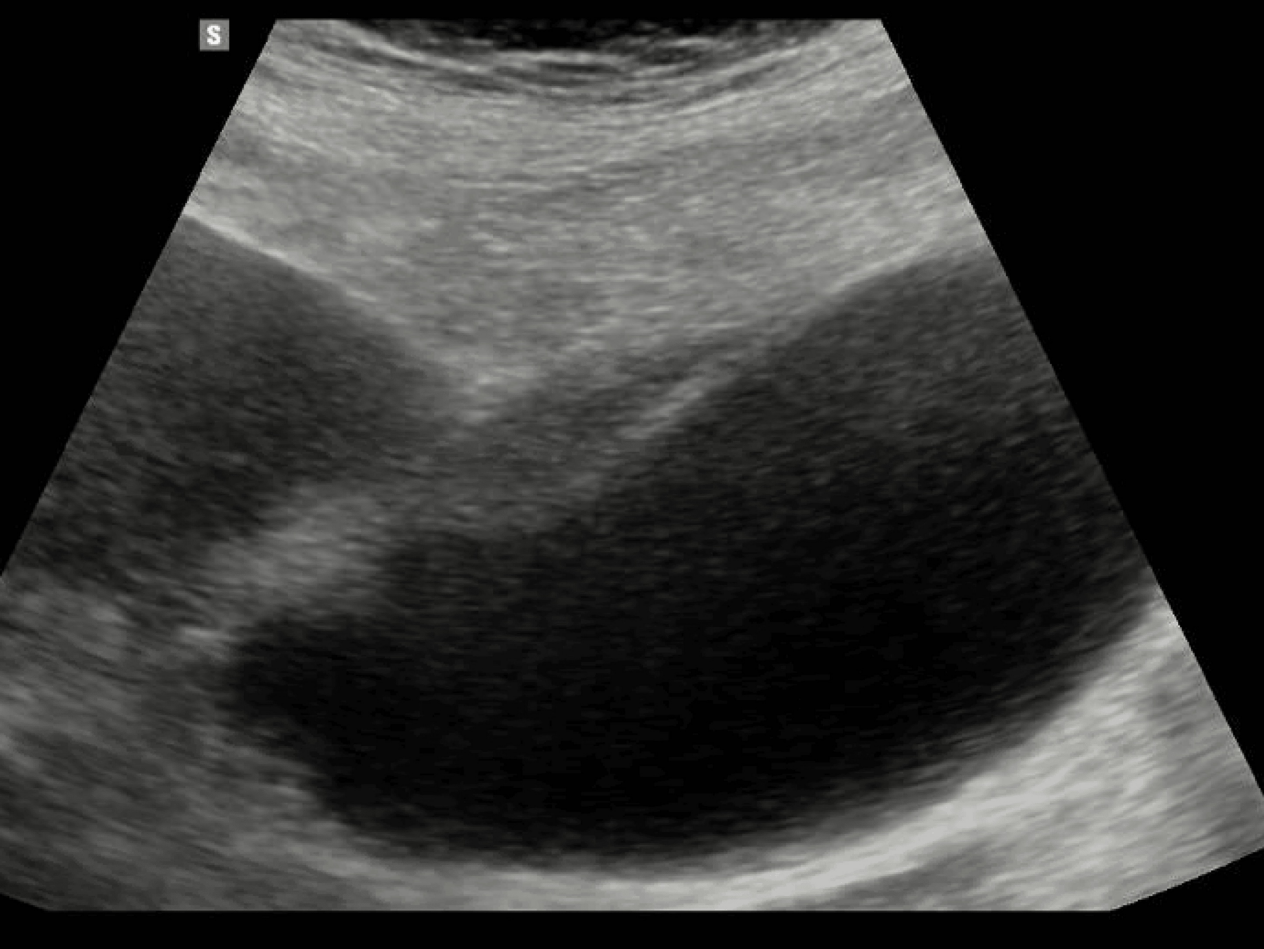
Ultrasound of the gallbladder showing a distended gallbladder. Symmetrical wall thickening of 8 mm with mild vascularity and evidence of pericholecystic fluid was noted. There was marked focal tenderness over the gallbladder. No gallbladder calculi were seen.
